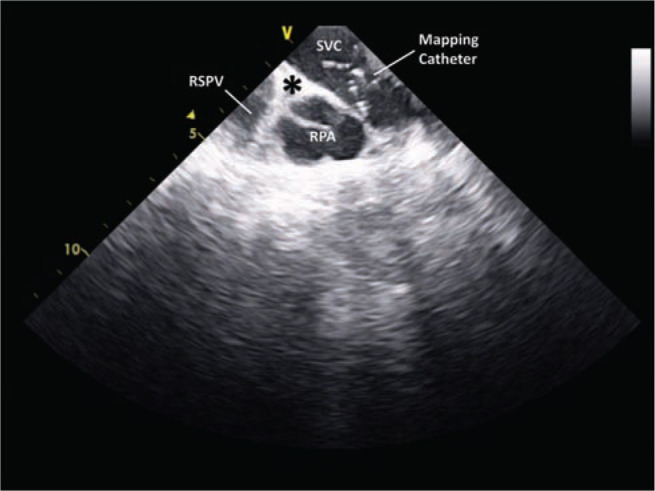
The superior vena cava-right atrium (SVC-RA) junction (marked by an asterisk) at the inferior border of the right pulmonary artery (RPA) on intracardiac echocardiography. The right superior pulmonary vein can be seen inferiorly to the RPA. A multi-electrode mapping catheter is present in the SVC, just above the AVC-RA junction.
